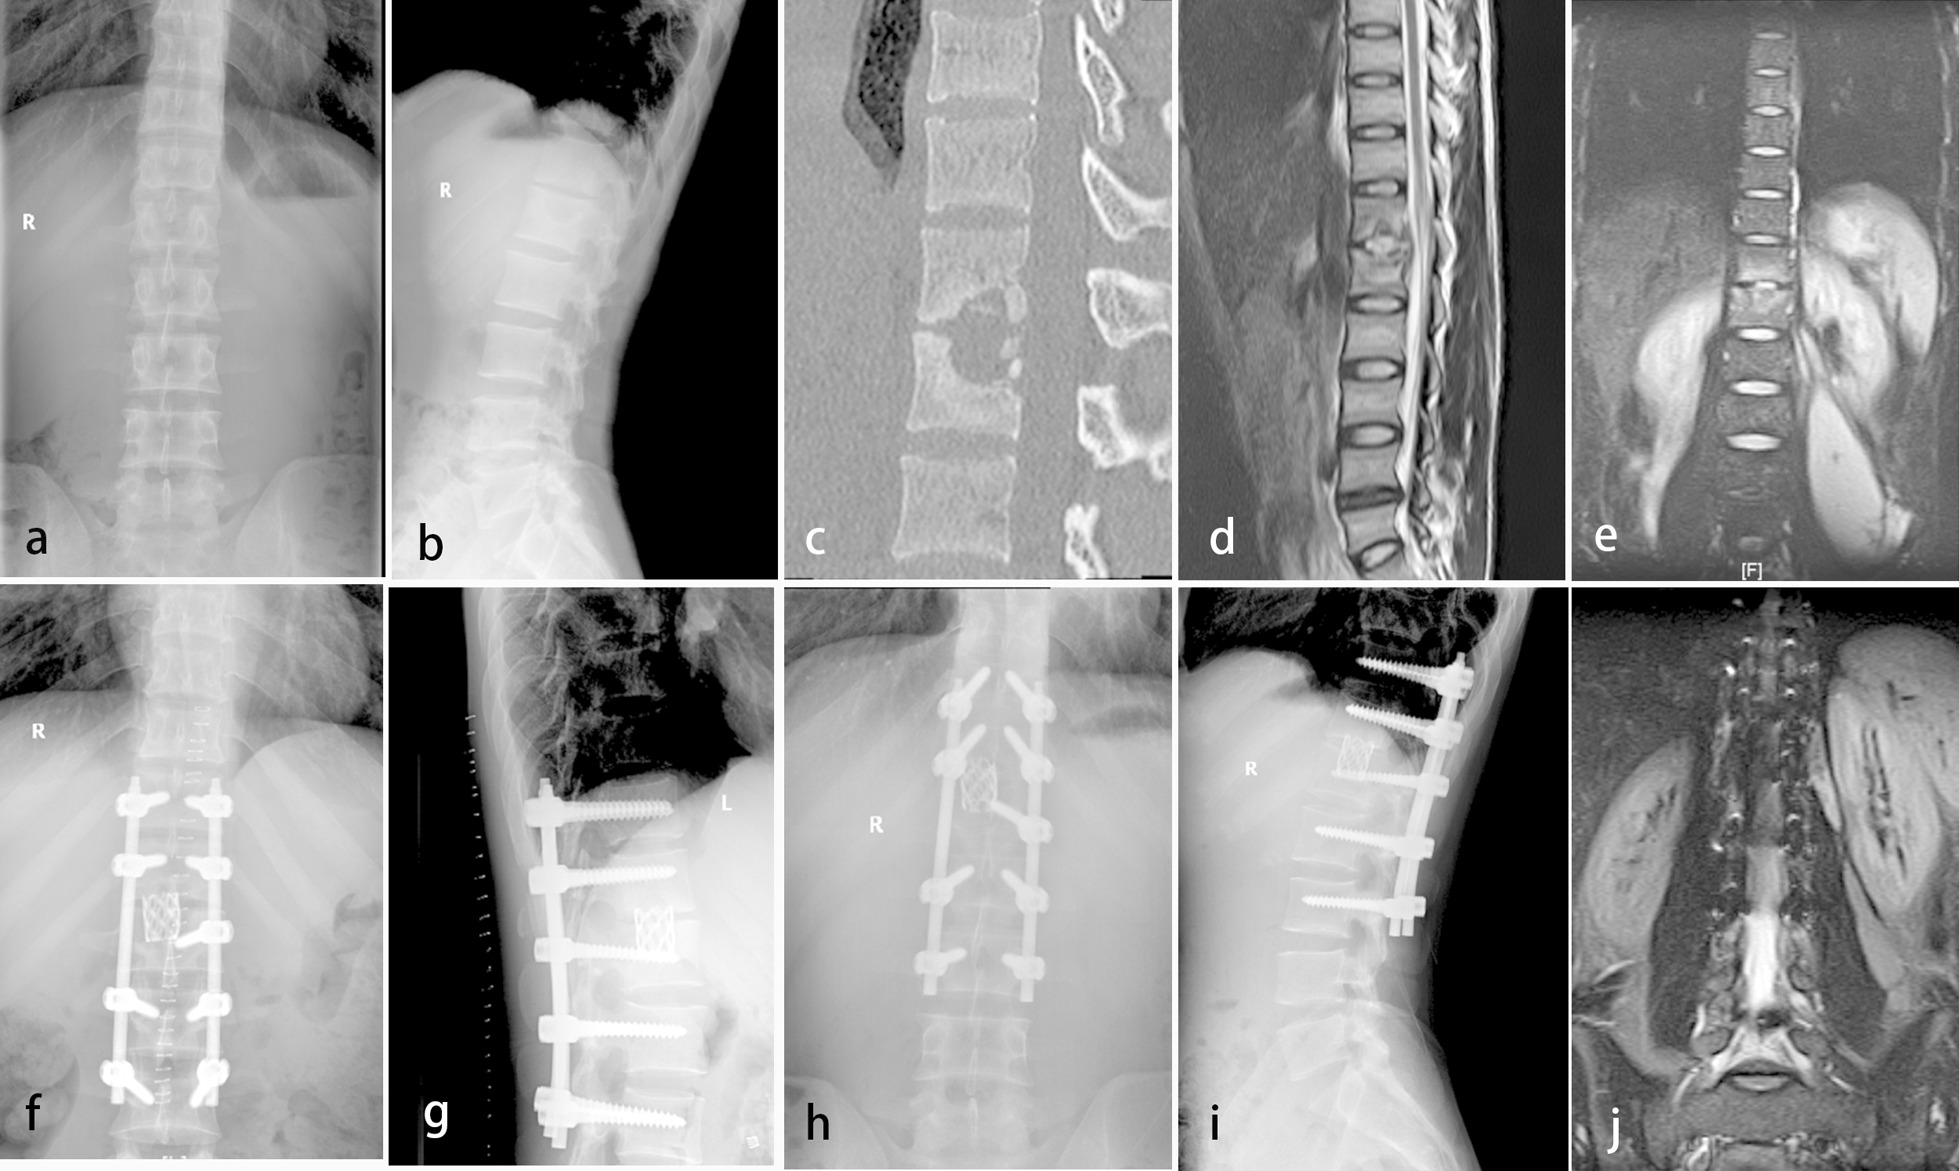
A 28-year-old male patient with thoracolumbar spinal tuberculosis accompany with unilateral psoas abscess (L1-L2). Preoperative radiography (a and b), CT (c) and MRI (d and e) showed destruction of anterior column and psoas abscesses. Radiography (f and g) showed no screw loosening or rod fracture appeared three months after surgery. Radiography (h and i) showed bone fusion at the final follow-up. MRI (j) showed psoas abscesses disappeared at the final follow-up
